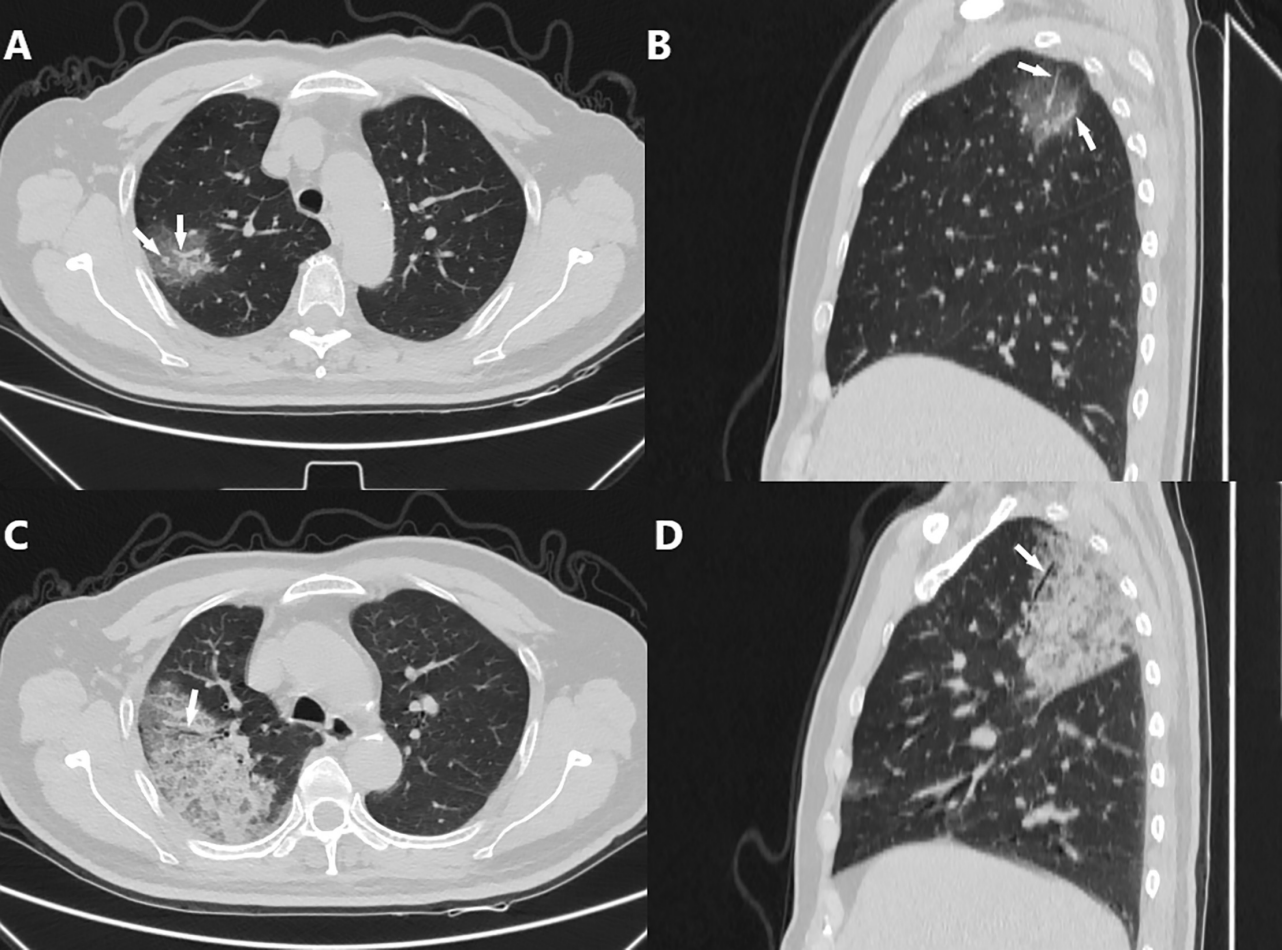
Typical CT manifestations 1. Chest CT images of a 68-year-old male who presented with cough and fever for 2 days. A and B. Axial CT scan (A) and sagittal reconstruction (B) shows a small area of approximately fan-shaped and subpleural GGO in the apical segment of the right upper lobe, with multiple small vascular enlargements (white arrows); the semiquantitative score = 2. C and D. Three days later, the lesion had progressed rapidly, and is displayed as a large area of fan-shaped/conical and subpleural crazy paving pattern with air bronchogram (white arrow); the semiquantitative score = 7.
